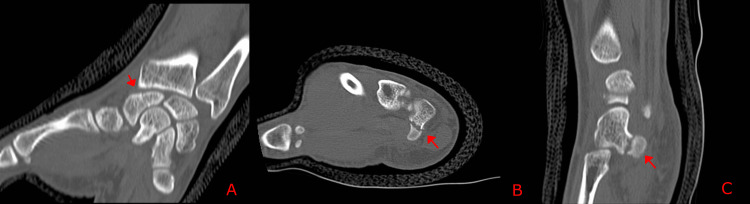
CT of the wrist at eight weeks demonstrating non-union fractures of the hook of hamate and scaphoid (A) Coronal CT images demonstrating a non-union scaphoid fracture (arrow). (B) Axial and (C) Sagittal CT images showing a non-union fracture of the hook of hamate (arrows). CT: computed tomography
